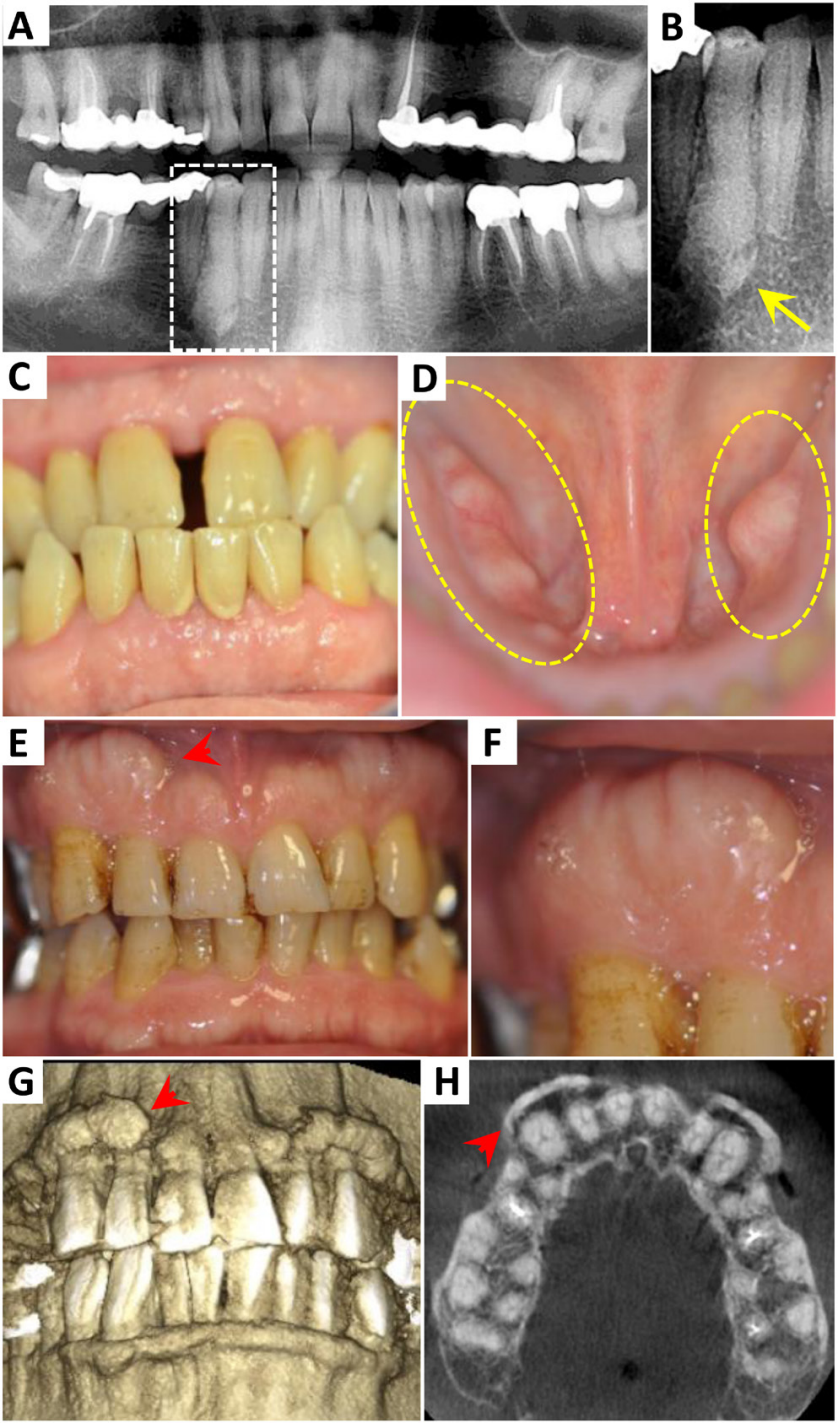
Acromegalic patients had frequent thick gingival biotype and oral bony outgrowths but no diffuse hypercementosis. (A) Orthopantomogram of an acromegalic patient recovering an isolated cementoma of the tooth 44 but no diffuse hypercementosis. (B) Enlargement of the dotted line delimited area in A, yellow arrow: cementoma. (C, D and F) Photographs of oral cavity of acromegalic patients showing: (C) thick gums without gingival hypertrophy nor periodontitis. Note the maxillary central diastema and occlusion disorder (class III); (D) presence of bilateral mandibular tori (circled in dotted line); (E) presence of vestibular bony outgrowths; (F) magnification of the bony outgrowth pointed by a red arrowhead in E; (G) 3D reconstruction from Cone beam CT (CBCT) of the same patient as in E; (H) CBCT slice through maxillary of the same patient as in E. Red arrow heads in E, G and H point to the same vestibular bony outgrowth, note the cortical bone enlargement H.
